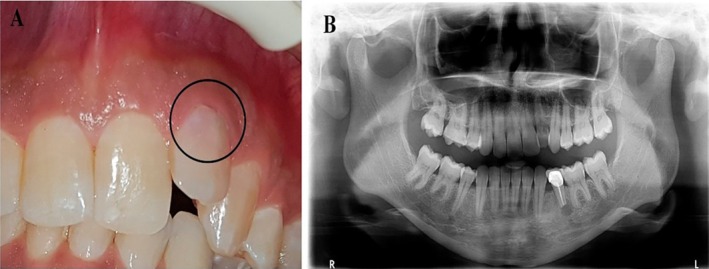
(A) Clinical photograph shows pink color at the cervical portion of the upper left lateral incisor. (B) Orthopantomogram showing an external root resorption of the maxillary left lateral incisor.
